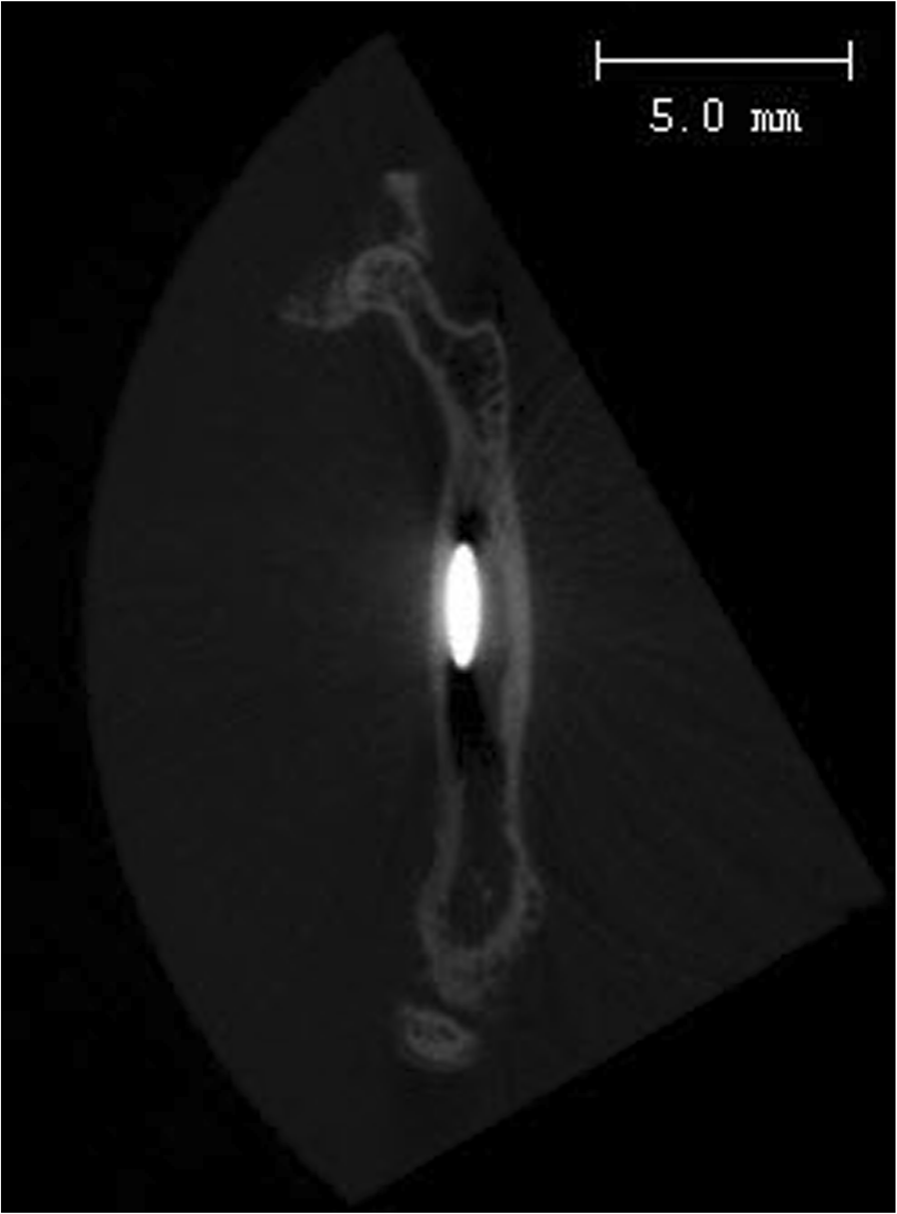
Coronal plane of a micro-computed tomography scan of the intramedullary compressed femur ten weeks postoperatively
